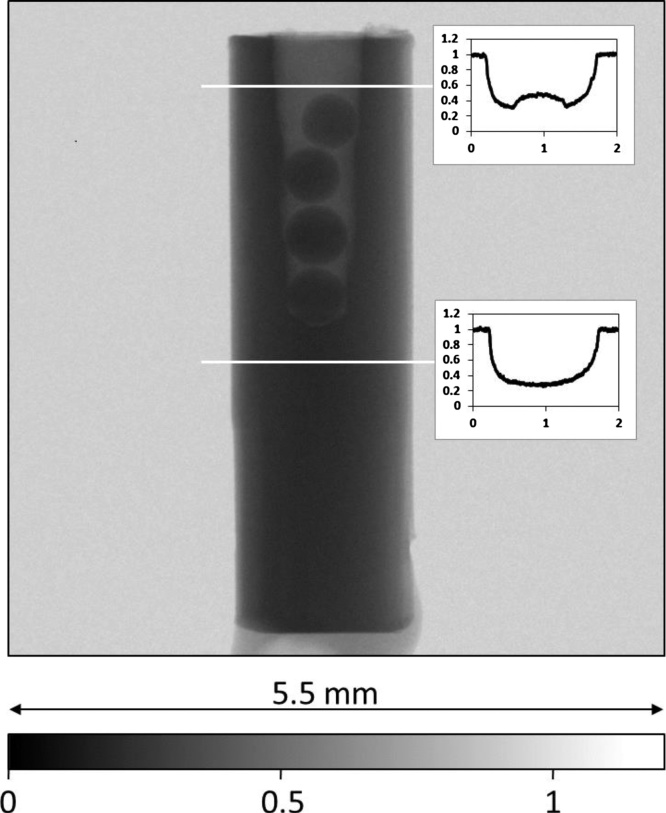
Corresponding neutron radiograph of the gold sample with artificial voids. The artificial pore space formed by 0.5 mm gold spheres placed in the drilled hole. The inserted transmission profiles clearly show clearly that inner pore space of the gold sample can be clearly revealed using neutrons.
